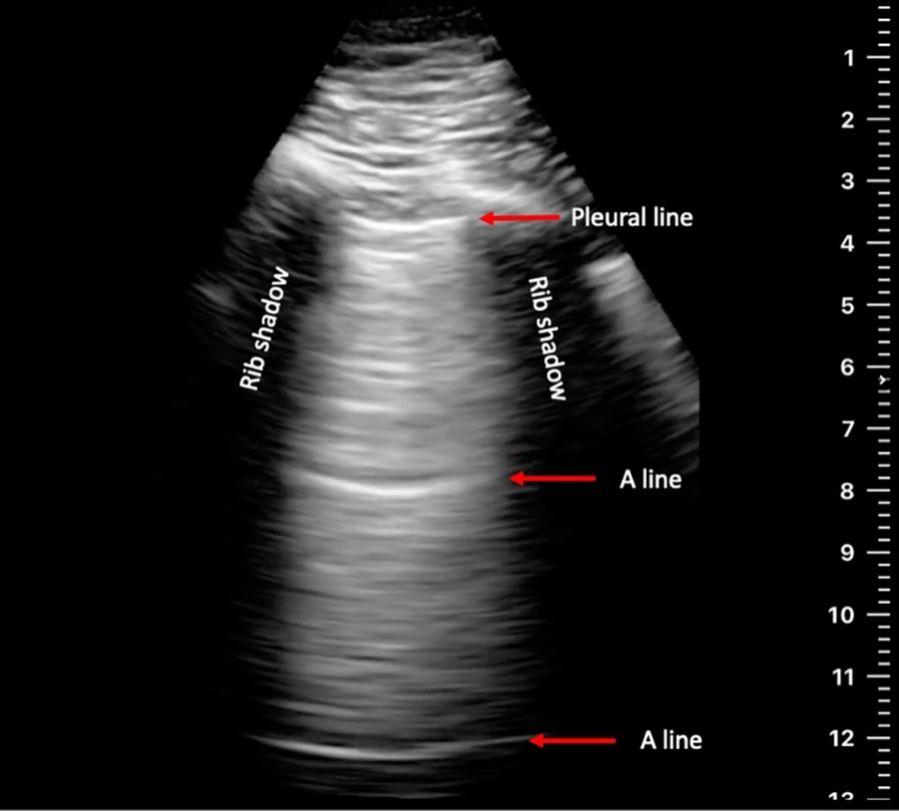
Representative image of a patient-performed ultrasound clip
